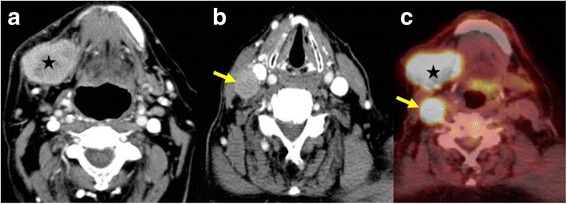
Patient with an undifferentiated cancer of the right submandibular gland. Contrast–enhanced CT scan a showing the solid, heterogeneously enhancing mass on the right side (star). CT scan at a slightly lower level b shows the solid metastatic lymph node (arrow). PET-CT image c showing the intense uptake of FDG glucose by the tumour (star) and the lymph node metastasis (arrow), both seen at the same level due to difference in the degree of neck flexion
